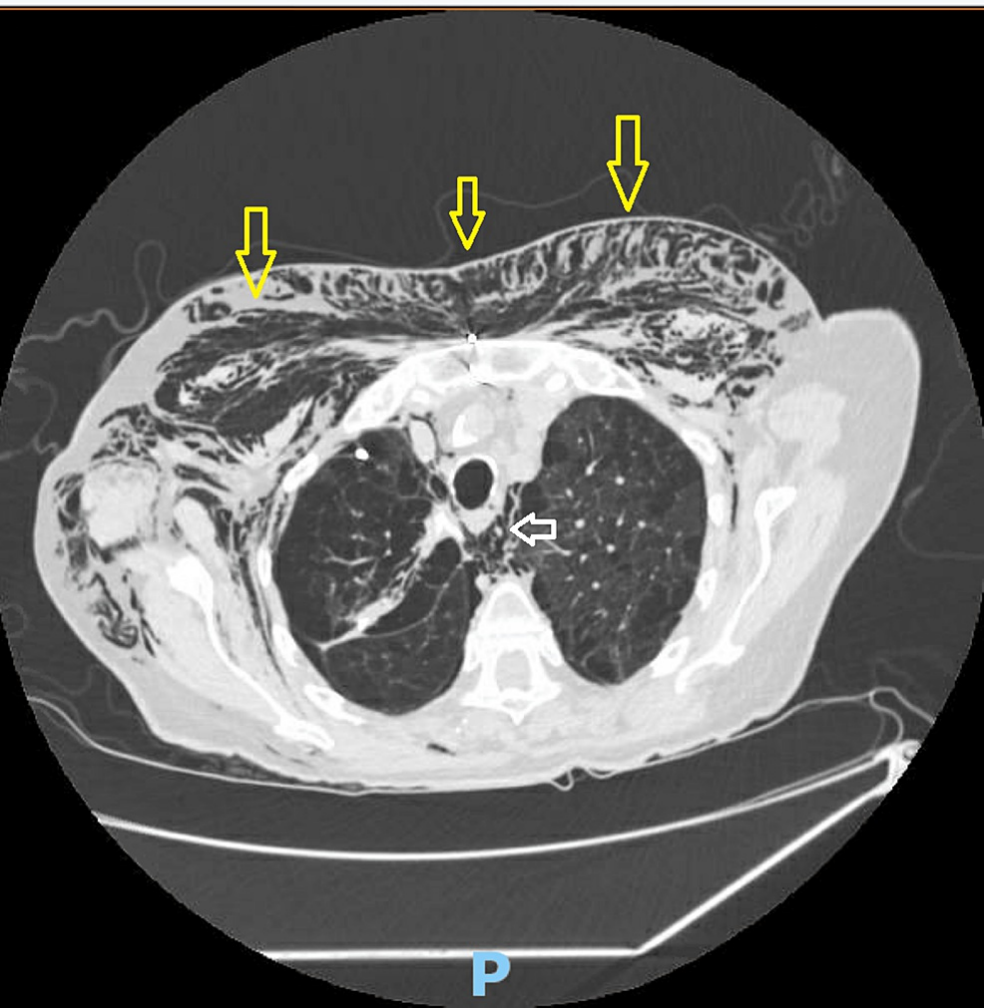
CT chest showing resolution of the pneumothorax. The image shows new pneumomediastinum (white arrow) and extensive subcutaneous emphysema involving the chest wall (yellow arrows).
